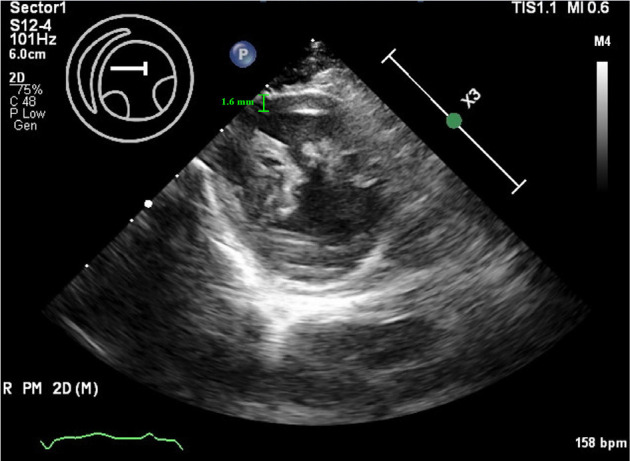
B-mode echocardiographic image showing focal thinning of the left ventricular free wall (green line)
